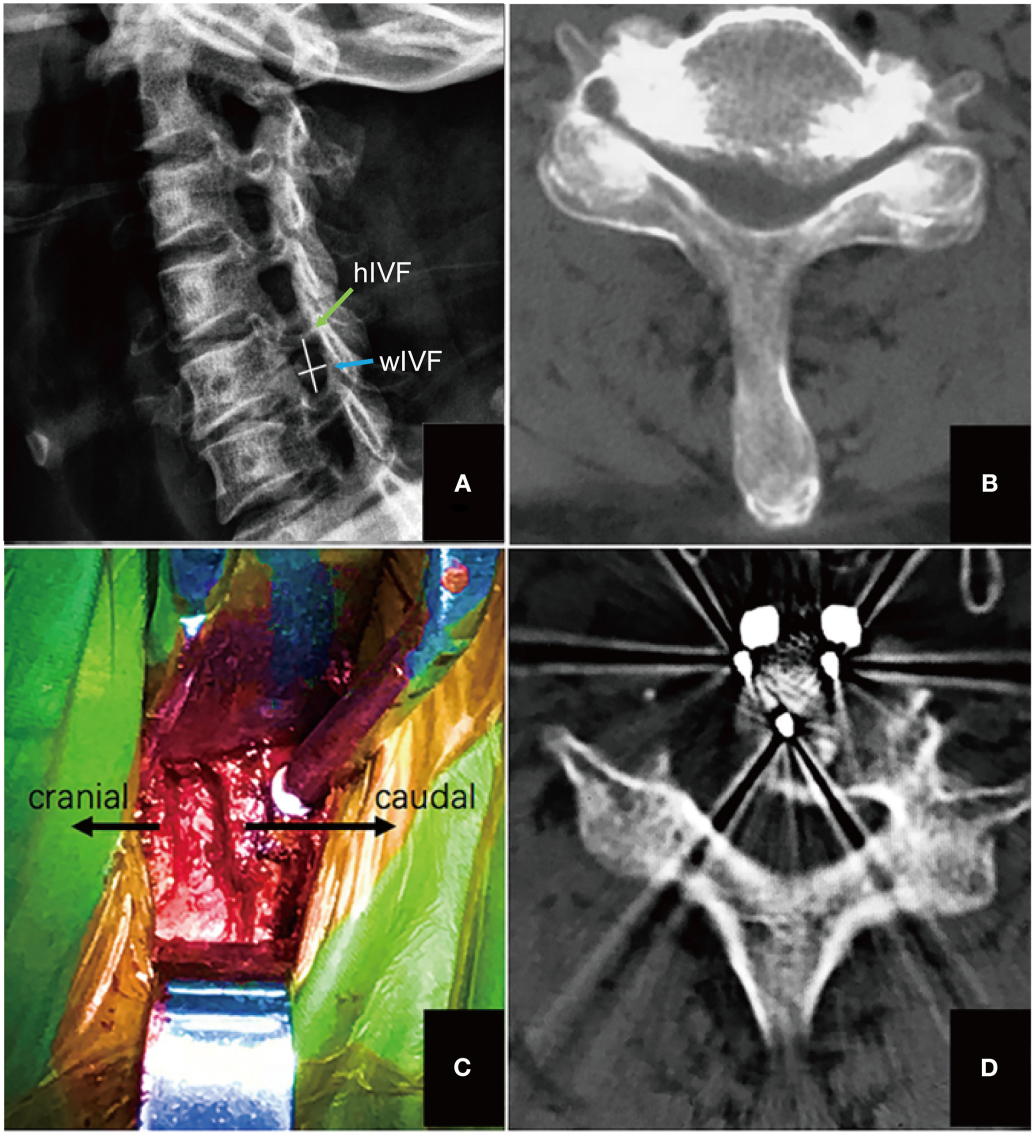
The process of performing UPR. (A) The width of intervertebral foramen (IVF) was measured by calculating the distance from the anterior medial point to the posterior medial point of the foramen (the transverse line) as width of IVF (wIVF), the height of IVF was measured by calculating the distance from the superior medial point to the inferior medial point of the foramen (the vertical line); (B) CT scan was used to confirm the hypertrophied uncinated process, meanwhile evaluating the anatomical relationship between vertebral artery and uncinated process; (C) the intraoperative view of surgical level after uncinated process was resected; (D) post-operative CT scan confirmed decompression of uncinated process osteophytes.
